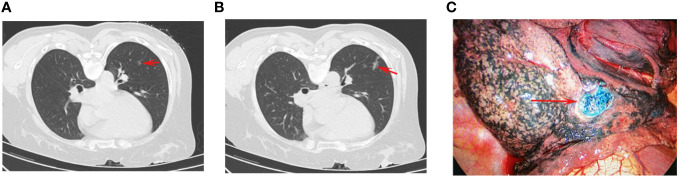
The procedures of CT-guided BSG localization. (A) The chest CT showed a GGN (arrow) at the left lower lobe. (B) The BSG (arrow) was injected near the GGN for localization. (C) The BSG (arrow) could be visualized during the VATS procedure.
